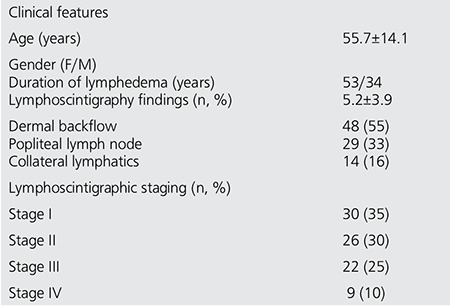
Demographic and lymphoscintigraphic characteristics of all patients (n=87)
